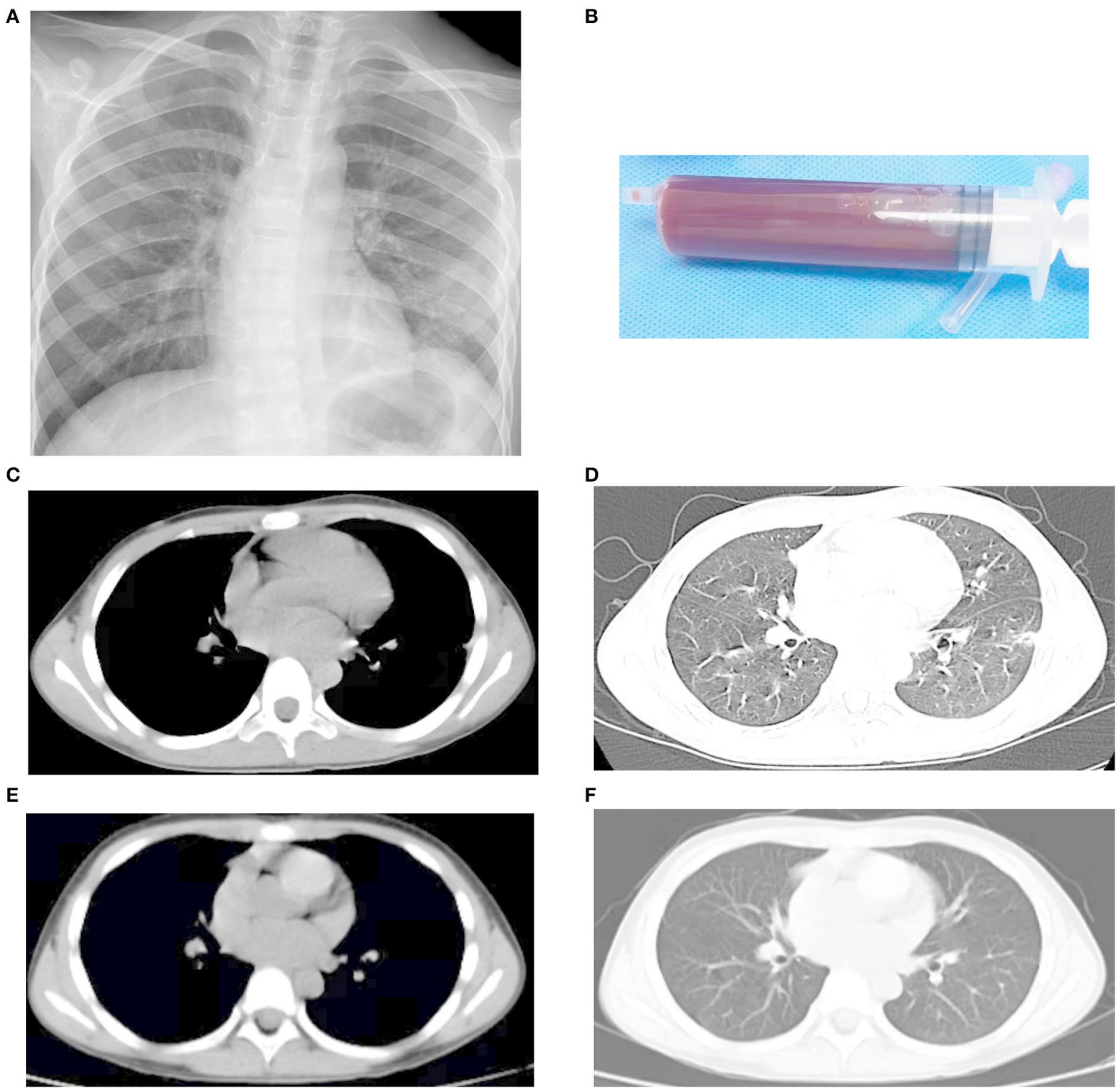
Radiological images after US-guided lung biopsy and appropriate treatment. (A) A repeated chest X-ray demonstrating partial resolution of the mass on hospital day 8. (B) Reddish-brown purulent pleural fluid. (C,D) A repeat chest scan confirming obvious resolution of the lung nodule on hospital day 20. (E,F) Follow-up CT after 3 months showing nodule resolution without any residual lesion.
